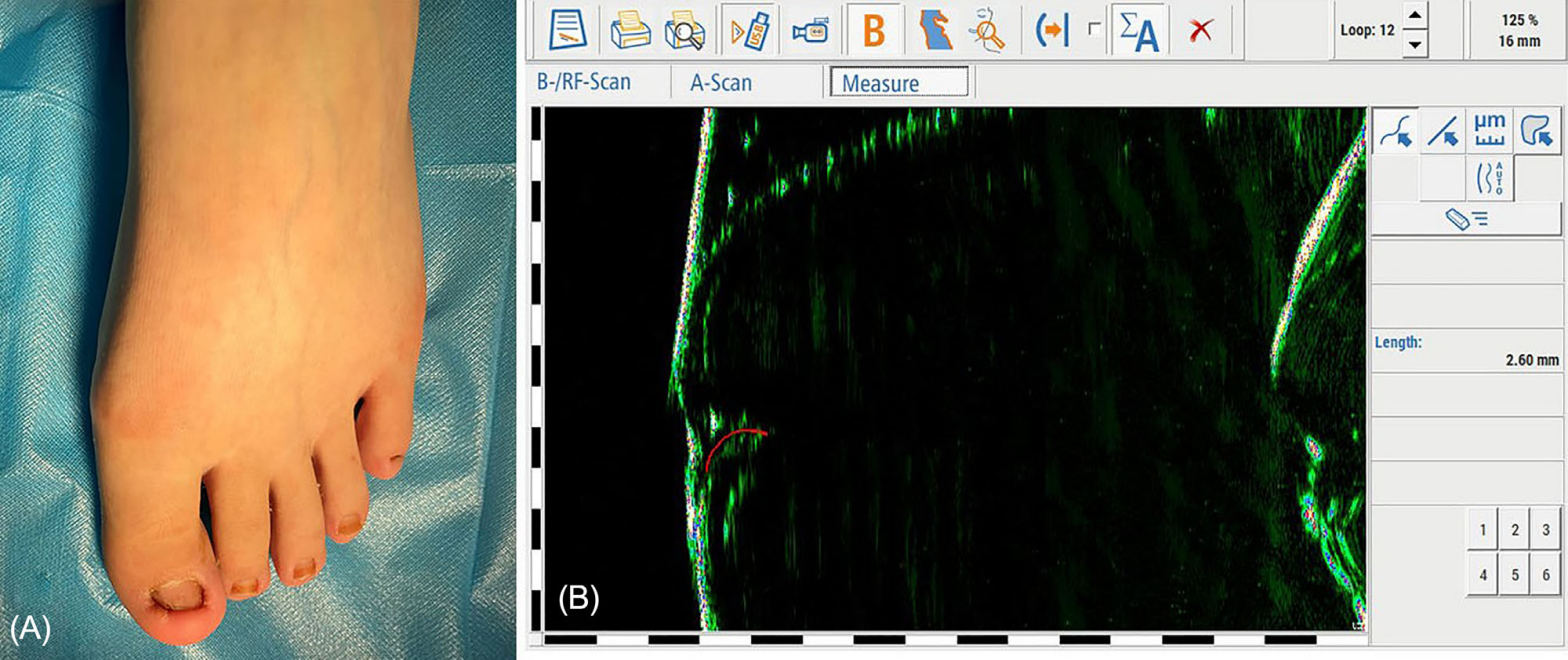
The use of high‐frequency ultrasonography (HF‐USG) overcomes the interference of the lateral nail groove and produced granulation tissues. (A) In the case of the plicated nail type of pincer nail with hypertrophic lateral walls similar to the ingrown nail. (B) D2 of the HF‐USG curvature index (2.6 mm) the length of the nail tip covered with hypertrophy of the lateral and distal nail folds under HF‐USG (red curve).
